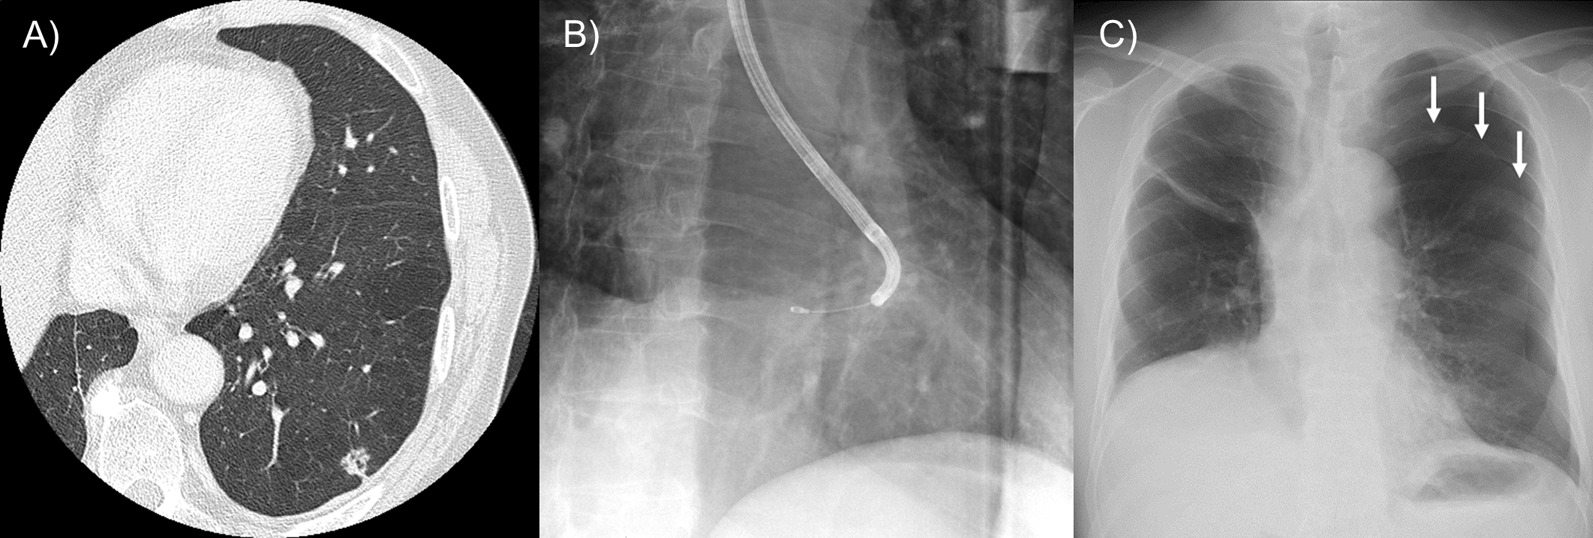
Representative case 1 of a patient who developed pneumothorax after cryobiopsy. A Computed tomography image showing a 12.4-mm solid nodule in the left lower lobe adjacent to the costal pleura. B The right anterior oblique view of the X-ray fluoroscopic image during cryobiopsy. The positional relationship between the pleura and cryoprobe could not be confirmed. C Chest plain radiograph acquired after the procedure revealed left pneumothorax (arrows)
